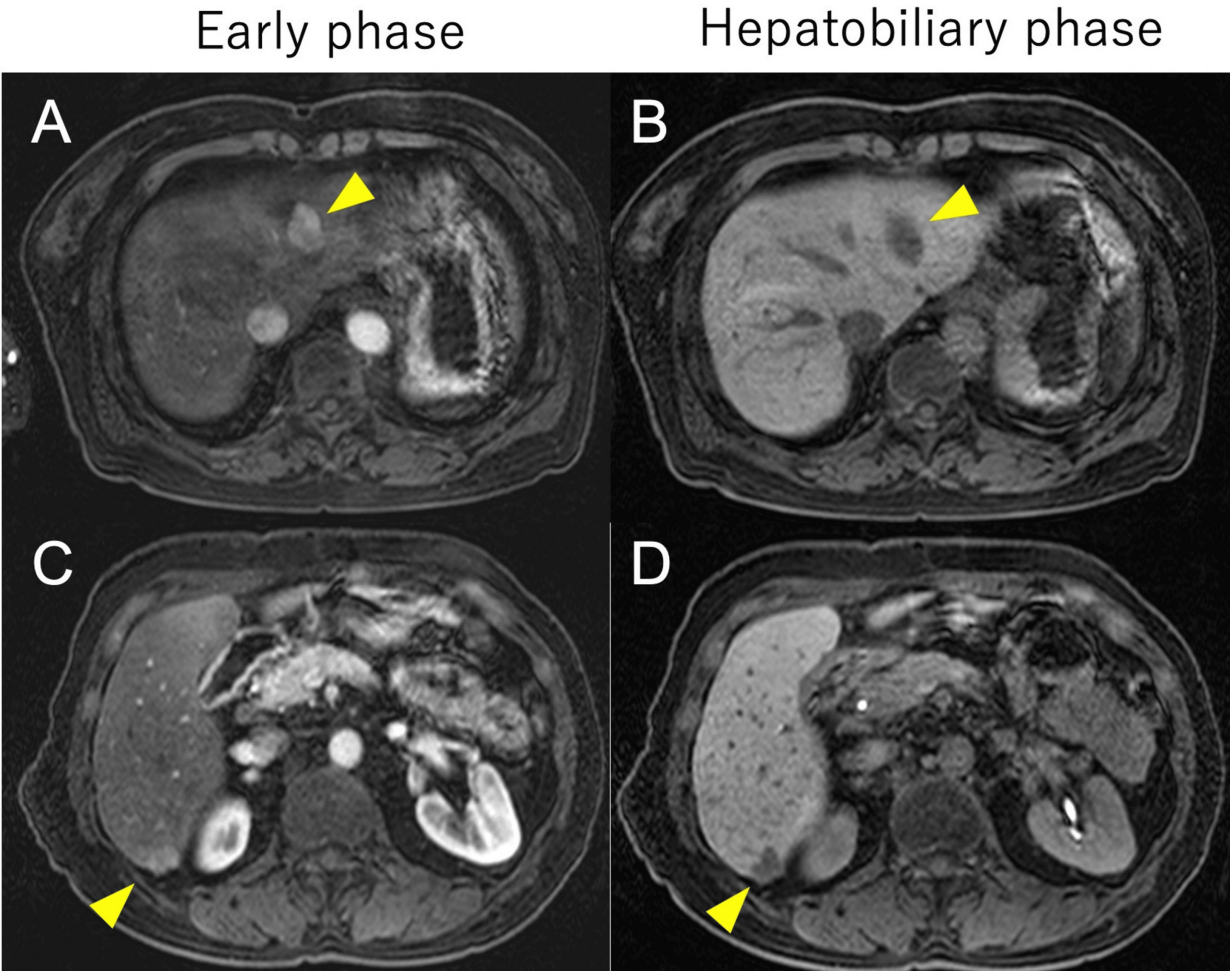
Gadoxetic acid-enhanced MRI (EOB-MRI) of the liver (A, C) Early arterial phase images showing hyperintense enhancement of the lesions in segment 2 (A, yellow arrowhead) and segment 6 (C, yellow arrowhead) of the liver. (B, D) Hepatobiliary phase (20 minutes post-contrast) demonstrating hypointensity of the lesions in segment 2 (B, yellow arrowhead) and segment 6 (D, yellow arrowhead) against the background of enhancing liver parenchyma. Both lesions demonstrate enhancement patterns often associated with hepatocellular carcinoma, showing hyperintensity in the early arterial phase and hypointensity in the hepatobiliary phase compared to the surrounding liver parenchyma.
